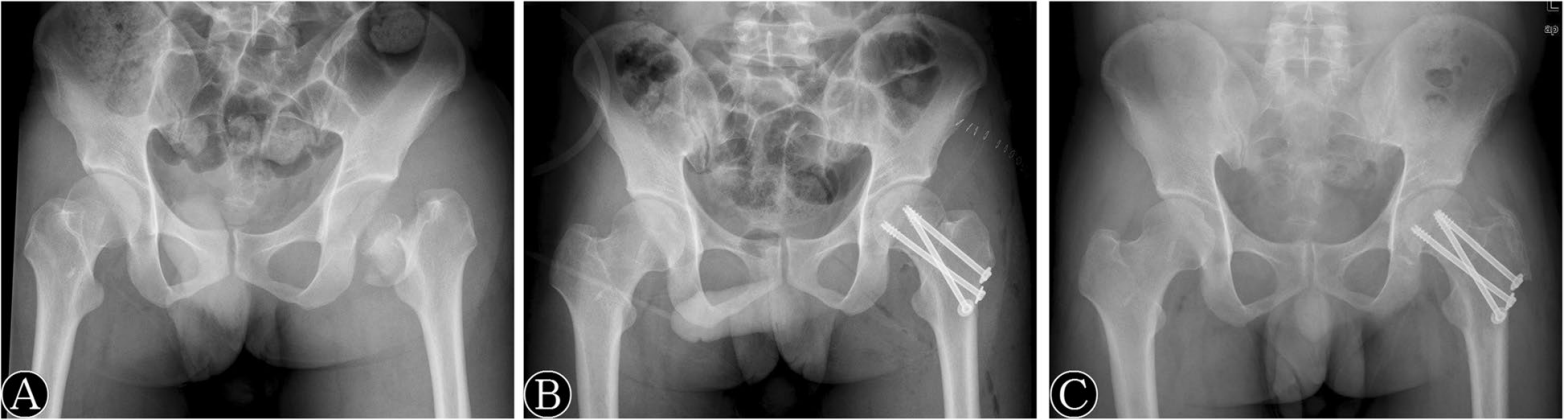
A-35-year-old man with left Pipkin type III fracture. A. Preoperative radiograph. B. Postoperative radiograph. C. Radiograph at 4 months demonstrating a bony union
